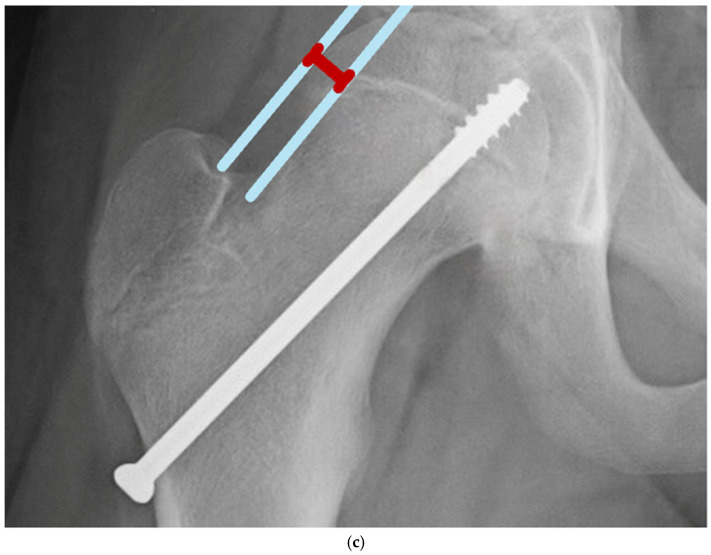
(a) The Southwick angle, a common measurement to determine the severity of a slip, was measured on the frog-leg lateral radiographs as the angle between a line drawn perpendicular to the line between the anterior and posterior tips of the physis, and a line along the axis of the femur. (b) The alpha angle was defined as the angle between two lines drawn from the centre of the femoral head, with one line being drawn longitudinal to the femoral neck and the other line being drawn to the point where the femoral head or neck breaks the circle of the femoral head anteriorly on the frog-leg lateral radiograph. (c) Displacement from Klein’s line was measured as the distance between two parallel lines drawn on the AP radiograph. The first was along the superior border of the femoral neck, and the second along the superolateral margin of the epiphysis.
